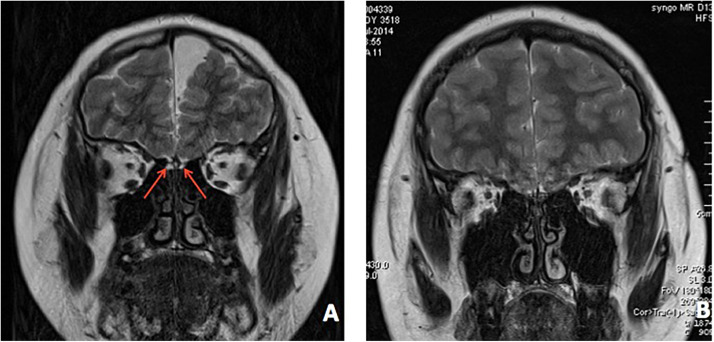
Brain MRI of both patients. (A) MRI revealed bilateral dysplasia of olfactory bulb, tract, and sulcu in patient 1. (B) MRI revealed bilateral dysplasia of olfactory bulb, tract, and sulcu in patient 2.
